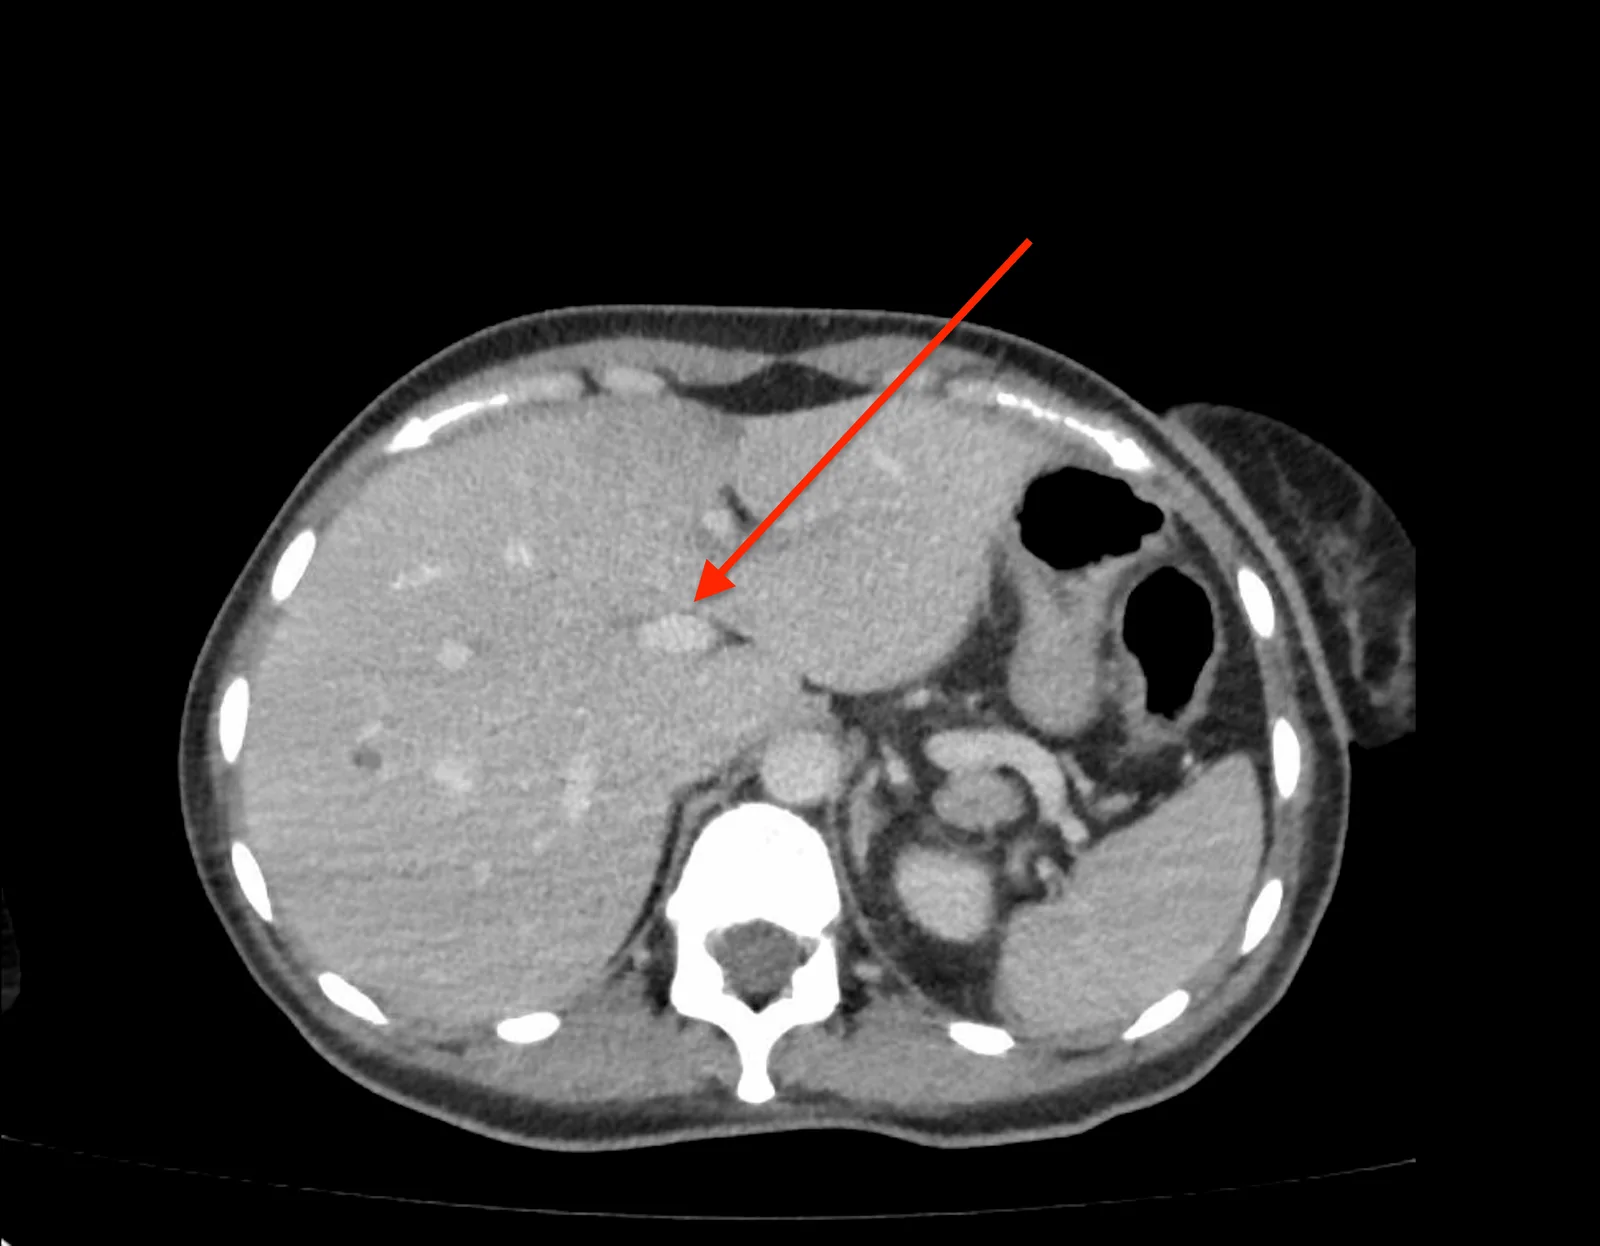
Contrast-enhanced computed tomography (CT) abdomen demonstrating hepatic septic embolus Contrast-enhanced CT abdomen demonstrating a hypodense lesion within the right hepatic lobe (arrow), suspicious for hepatic septic embolus (or hepatic abscess) in the setting of disseminated infection. Additional small hypodense lesions were seen in both hepatic lobes.
